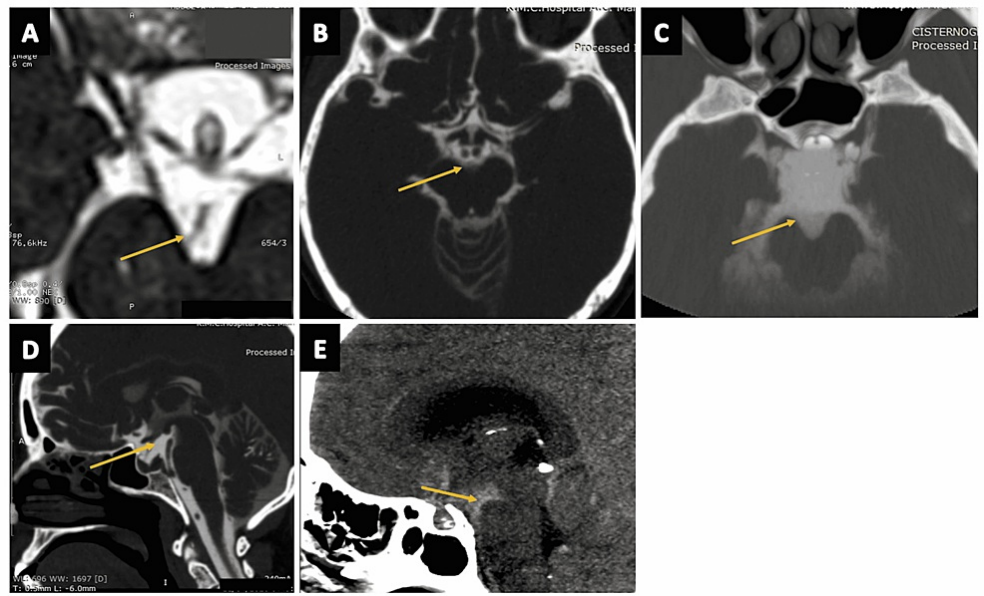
The cisterna interpeduncularis (interpeduncular cistern). (A) A zoomed-in portion of an axial section of the routine three-dimensional (3D) constructive interference in steady state (CISS) MRI sequence highlighting the interpeduncular cistern (arrow). (B), (C), and (D) show the interpeduncular cistern (arrows) in the axial, oblique-axial, and sagittal sections of CT cisternography, respectively, in a case of suspected CSF rhinorrhoea. (E) The interpeduncular cistern (arrow) as seen on the sagittal plane of CT brain (positive contrast) in a case of subarachnoid hemorrhage (SAH).
